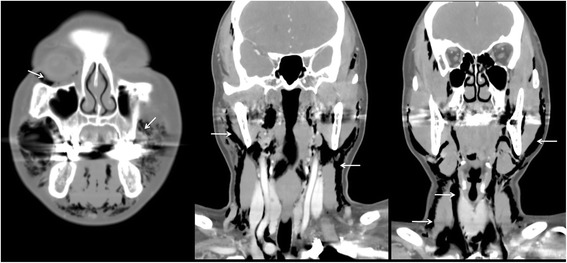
Paranasal sinus computed tomography (PNS CT [coronal view]) of emphysema in the retromandibular, visceral, and retropharyngeal spaces (arrows)
